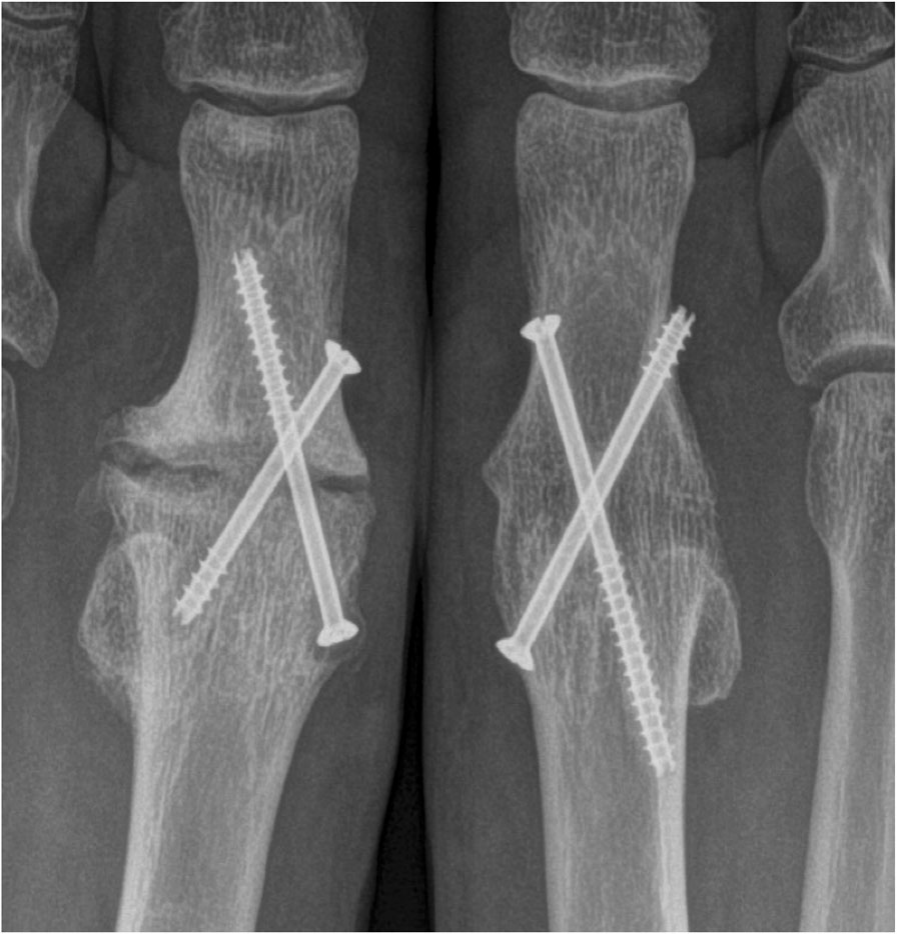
Detail of a postero-anterior standing radiograph of both feet following first metatarsophalangeal joint arthrodesis, in a 48 year-old female. Fusion of the hallux was achieved on the right foot but not on the left. Note the radiolucent line surrounding the implants in the metatarsal end of the fusion, and the periosteal reaction around the screw head. Revision surgery showed the presence of coagulase-negative staphylococci
